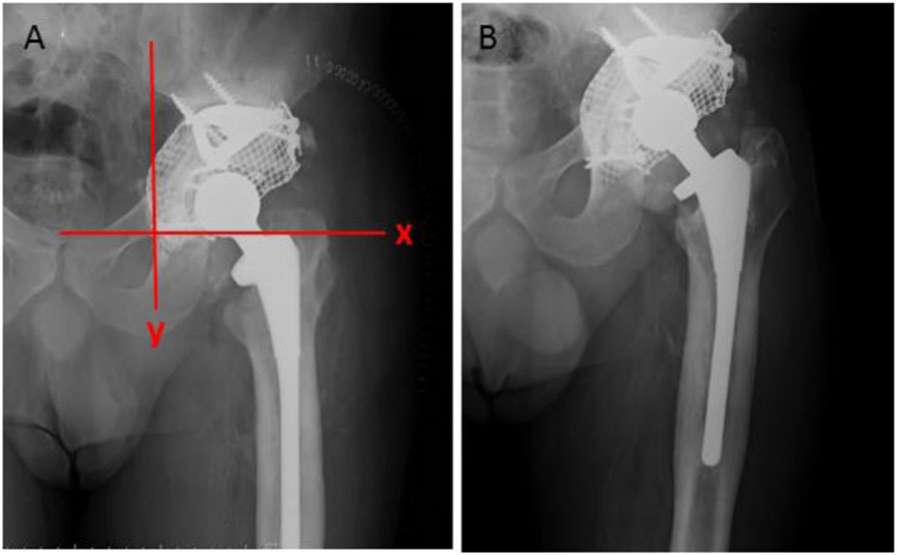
Case 4: (a) Radiographs immediately postoperatively and 6 years post-surgery (b) showing the acetabular component position using the method described
